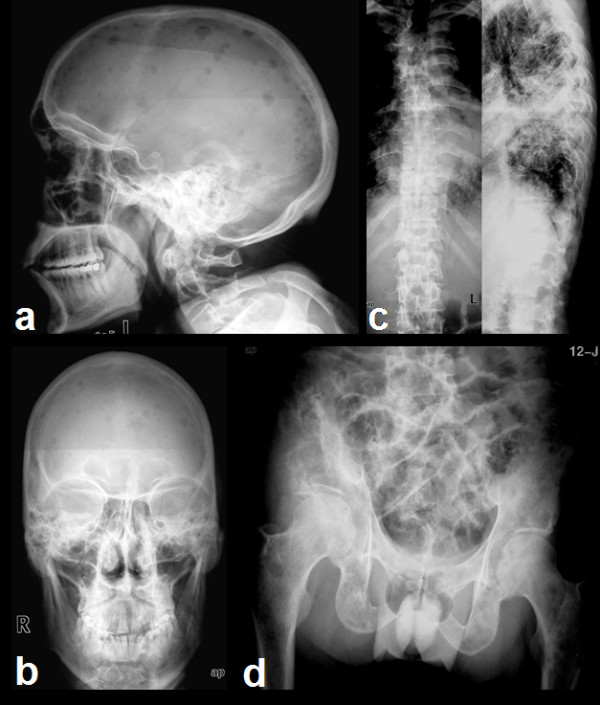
Radiographs of the skull (a, b), spine (c), and pelvis (d) showing multiple variably sized lytic lesions affecting all visualized bones.
